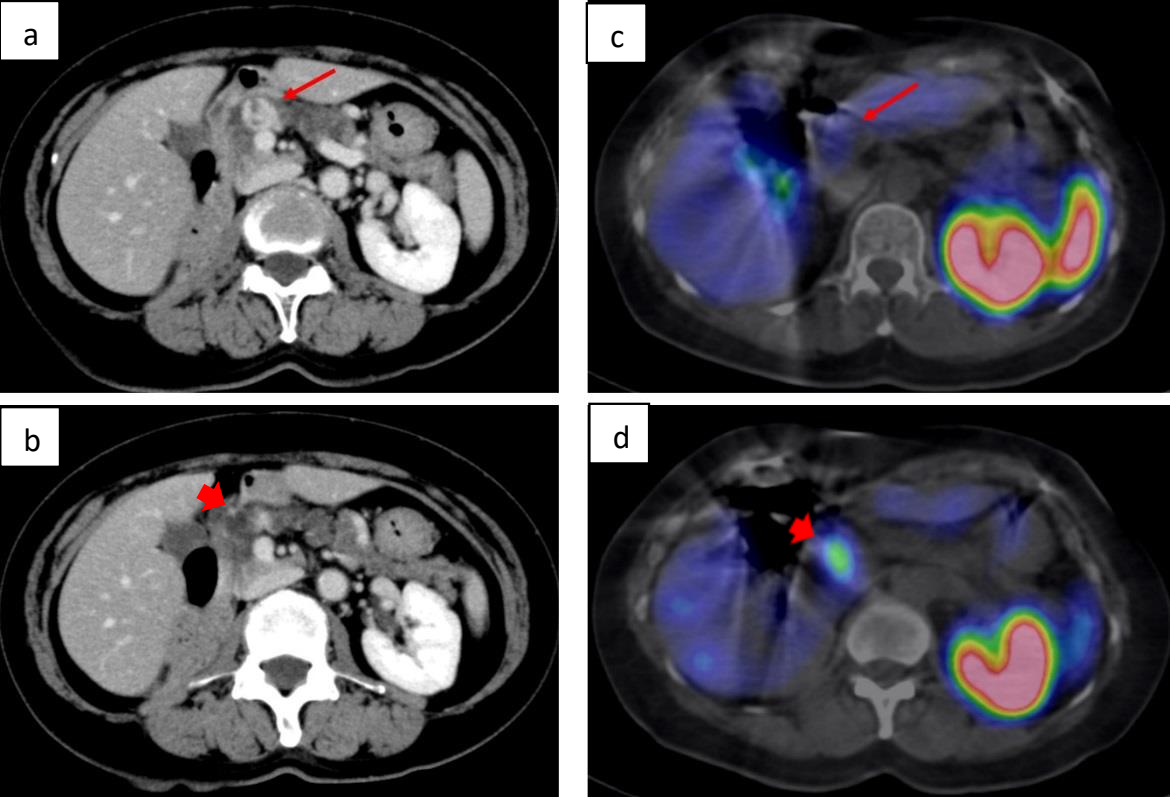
A female patient in her 60s showed a hypervascular mass lesion (4a. arrows) in the pancreatic head on contrast-enhanced CT, which occurred following right nephrectomy, but NEN was considered in the differential diagnosis, and SRS was performed. SRS-SPECT/CT (4d. arrowhead) showed increased accumulation in the uncus of the pancreas head, which was misidentified for accumulation in a mass lesion. Indeed, mass lesions do not accumulate in SRS (4c. arrow), and sites of SRS accumulation (4d arrowhead) are not visible on CT (4b. arrowhead). The resected tumor was diagnosed as pancreatic metastasis from renal cell carcinoma
